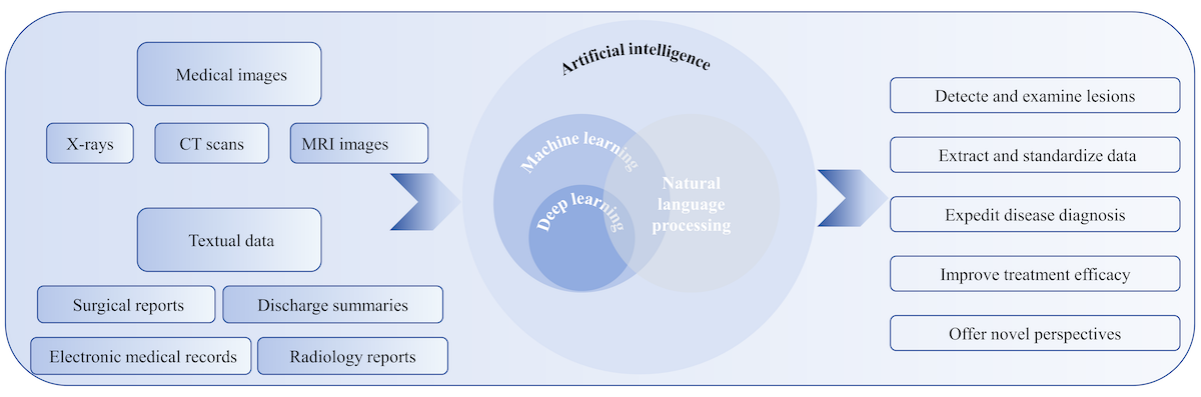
Types of AI and their application in the medical field. CT: Computed Tomography; MRI: magnetic resonance imaging.
